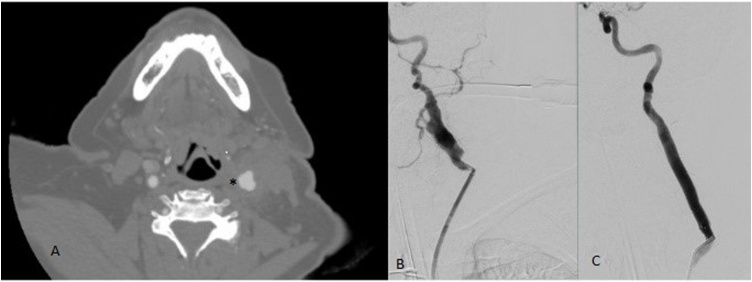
A. Computed tomogram angiography (CTA) showing evidence of left carotid pseudoaneurysm. B. Selective left carotid angiography showing the pseudoaneurysm extending to skall base. C. Successful deployment of left carotid sent graft with no evidence of extravasation.
